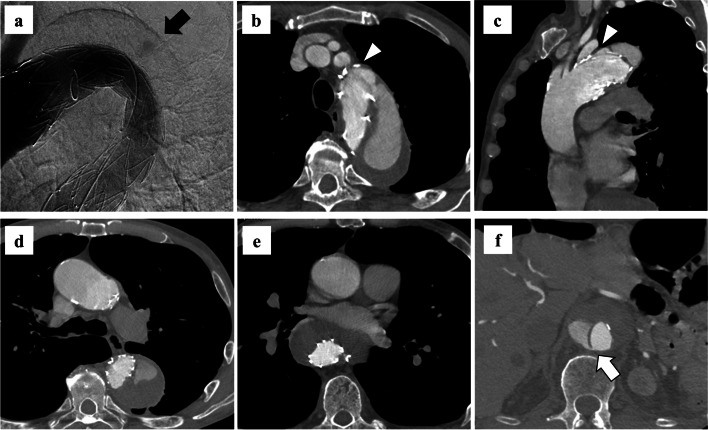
a Intraoperative digital subtraction angiography after deployment of the stent graft. The black arrow indicates endoleak. b–f Contrast-enhanced computed tomography after thoracic endovascular aortic repair. The false lumen remained open, and the diameter of the distal aortic arch was enlarged. b, c The white arrowheads indicate type Ia endoleak. d The maximum diameter of the distal aortic arch is 57 mm. e The true lumen compression is improved by thoracic endovascular aortic repair, but the stent graft remains slightly compressed. f The white arrow indicates a re-entry located above the celiac artery
